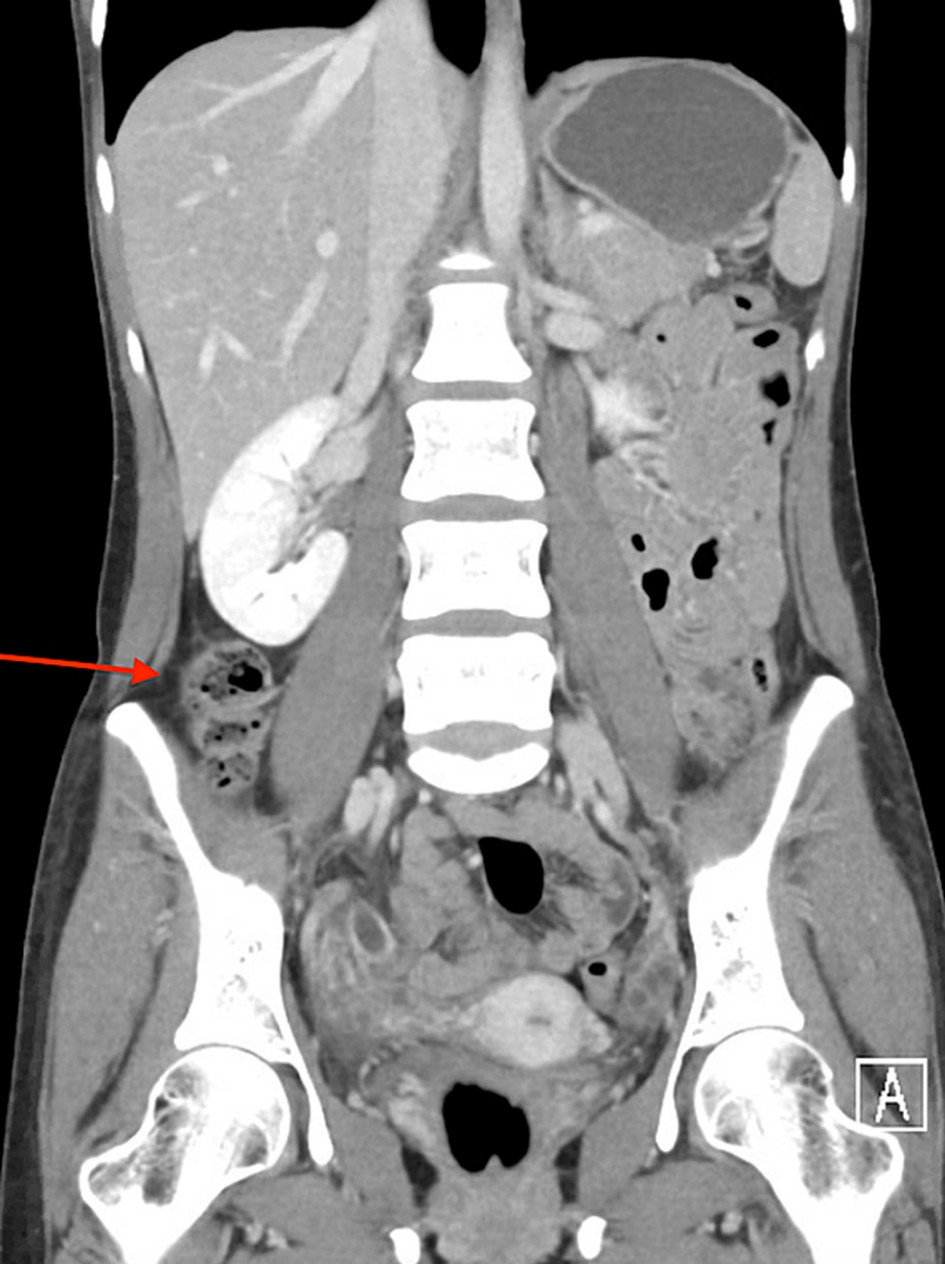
CT abdomen pelvis with contrast showing a dilated appendix (red arrow)
